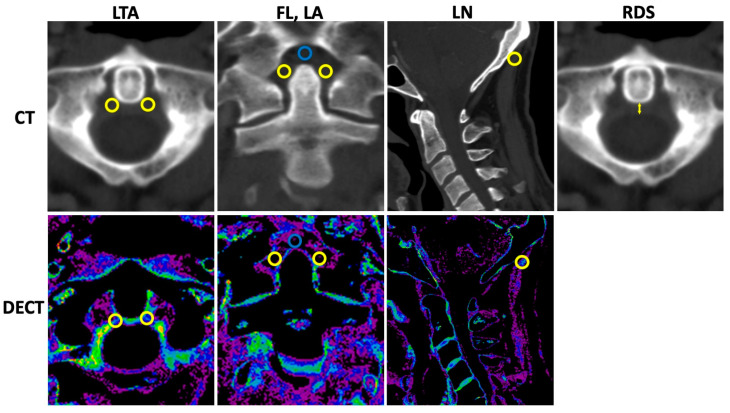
Measurement of collagen density and retro-odontoid soft tissue. CT = computed tomography, DECT = dual-energy CT, LTA = ligamentum transversum atlantis, FL = fasciculi longitudinalis, LA = ligamenta alaria, LN = ligamentum nuchae, and RDS = retro-odontoid soft tissue thickness. Upper row indicates CT with 135 kVp reconstructions. Lower row refers to DECT-based collagen maps. Region of interest (ROI) measurements were performed with predefined size of 9.5 mm^2^ for all measurements using the collagen density maps. In total, twelve ROI measurements were performed (six in 135 kVp images, six in collagen maps) as follows: LTA (two ROIs per reconstruction in axial orientation (left and right orientation, yellow ROIs); FL (one ROI per reconstruction in coronal orientation, blue ROI); LA (two ROIs per reconstruction in coronal orientation, yellow ROIs); and LN (one ROI per reconstruction in sagittal orientation, yellow ROI). Thickness of the retro-odontoid soft tissue (indicated by the yellow arrow) was measured using the 135 kVp CT reconstruction in axial orientation.
